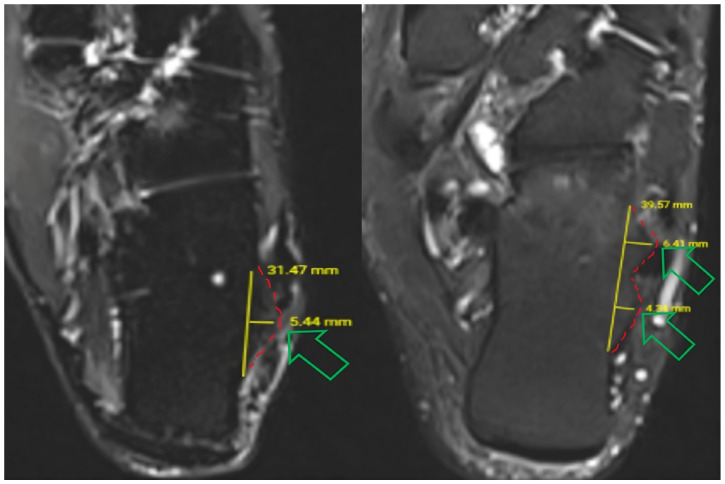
Axial MRI examples demonstrating peroneal tubercle height and anteroposterior length measurements (yellow lines) at the level of maximal prominence and the classification of single-convex (left) and double-convex (right) morphologies. The green hollow arrow on the left indicates the apex of a single-convex peroneal tubercle, while the two on the right indicate the apices of a double-convex peroneal tubercle morphology. The red dashed lines mark the upper boundary of the tubercles on both the right and left.
